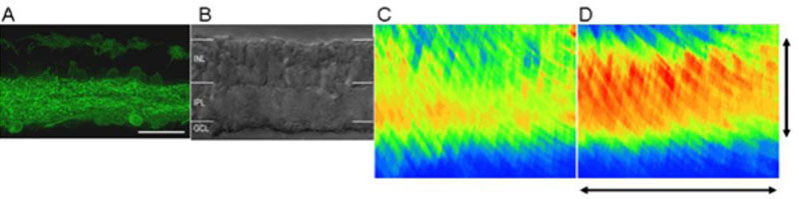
Vertical analysis of the retina. A: Numerous green fluorescent protein (GFP)-positive ganglion, amacrine, and horizontal cells can be seen in the cryostat section of a retina after a light-exposed magnetic resonance imaging experiment. B: Nomarski image of the same region shown in (A) reveals the absence of photoreceptors. Abbreviations are as follows: INL represents inner nuclear layer; IPL represents inner plexiform layer; GCL represents ganglion cell layer. The scale bar is equal to 25 μm in A and B. C-D: Pseudocolor linearized average 1/T1 maps of the central retina from uninjected (C) and virus injected (D) eyes, scaled vertically to approximately match the boundaries of (A) and (B). The pseudocolor scale was *blue* to *green* to *yellow* to *red*, which represents lowest (0.56 s^−1^) to highest (2.25 s^−1^) 1/T_1_ values. 1/T_1_ is directly proportional to manganese concentration [16]. The vertical scale bar is equal to 130 μm, and the horizontal scale bar is 0.6 mm and applies to C and D.
